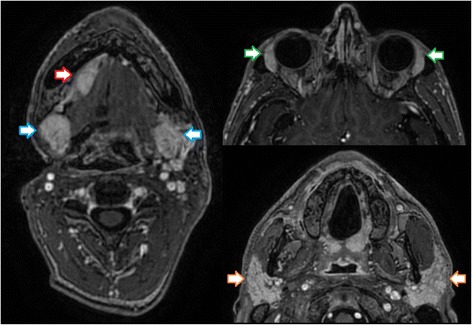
T1-weighted MRI in December of 2016 showing signs of inflammation with marked swelling of salivary glands (sublingual, red arrow; submandibular, blue arrows; parotids, orange arrows) as well as lacrimal glands (green arrows)
